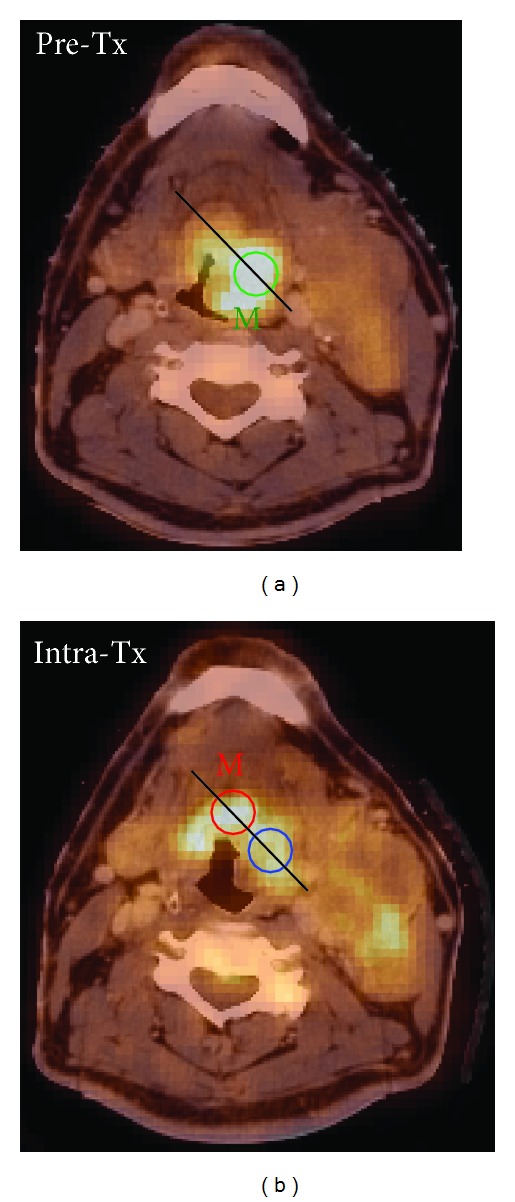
Change in the distribution of FDG uptake during treatment. The PET/CT images of Pre-Tx (a) and Intra-Tx (b) are of a patient with a base of tongue primary tumour. Two circular ROIs of 15 mm diameter are centered at the maximum uptake points on both Pre-Tx (green) and Intra-Tx (red) images denoted by “M.” An additional 15 mm diameter circular ROI is placed on the Intra-TX image (blue) in a position judged to correspond to the same anatomical location as the ROI as in the Pre-Tx. The FDG uptake profiles along the black lines connecting the two Intra-Tx ROIs are shown in Figure 2.
